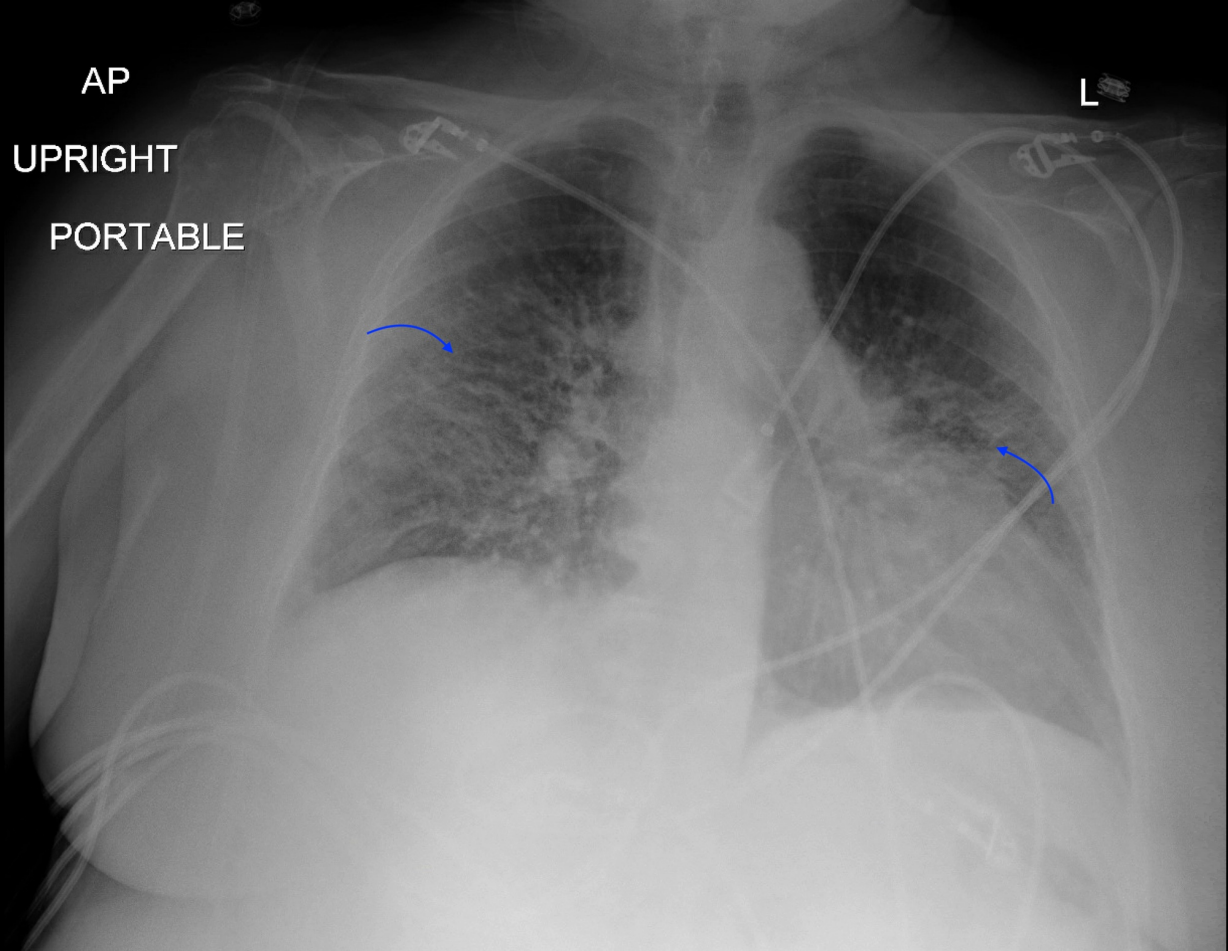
Chest X-Ray Bilateral patchy asymmetrical opacities without cardiomegaly or pleural effusions. AP: anterior-posterior; L: left.
